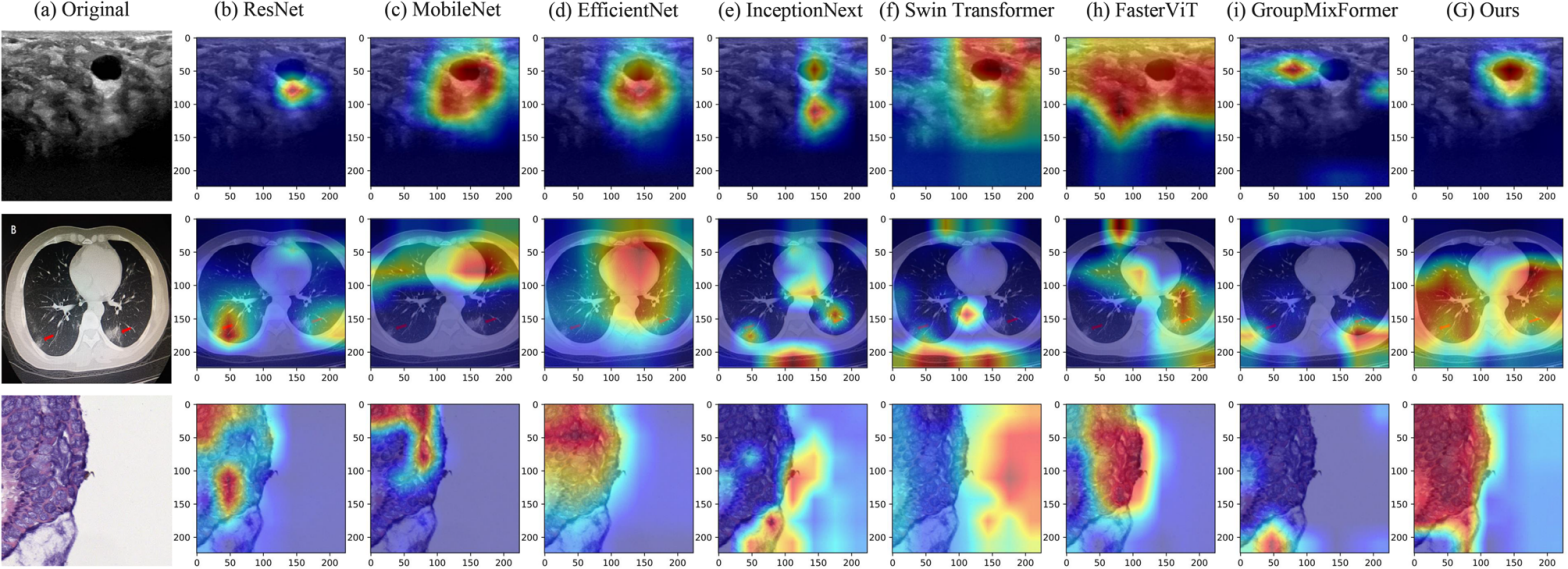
Grad-CAM visualization results for different comparison models on the BUSI, COVID19-CT, Chaoyang datasets.
